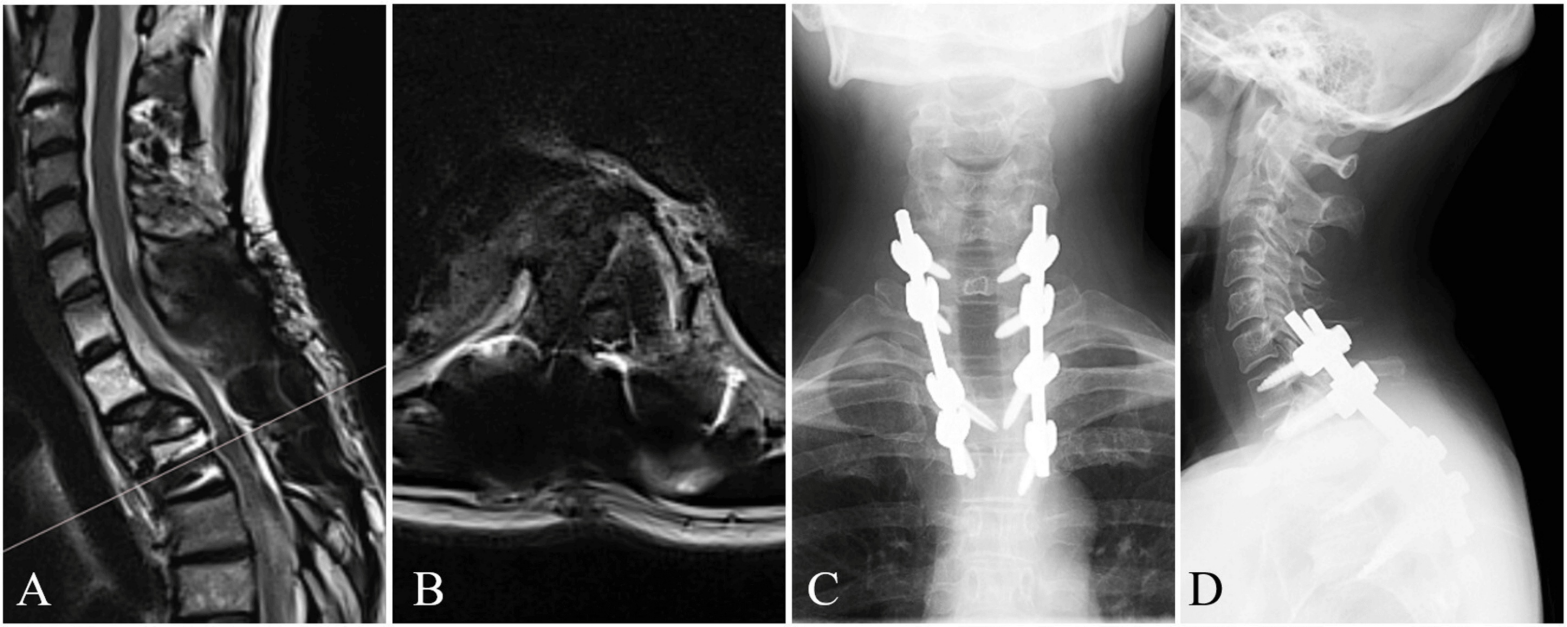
Magnetic resonance imaging and plain radiographs (A) T2-weighted sagittal image and (B) T2-weighted axial image at T3 obtained six months before presentation showed no abnormalities at the surgical site and no signs of seroma. (C, D) Plain radiographs at presentation. The spinal alignment was preserved and no radiographic evidence of instability was observed.
